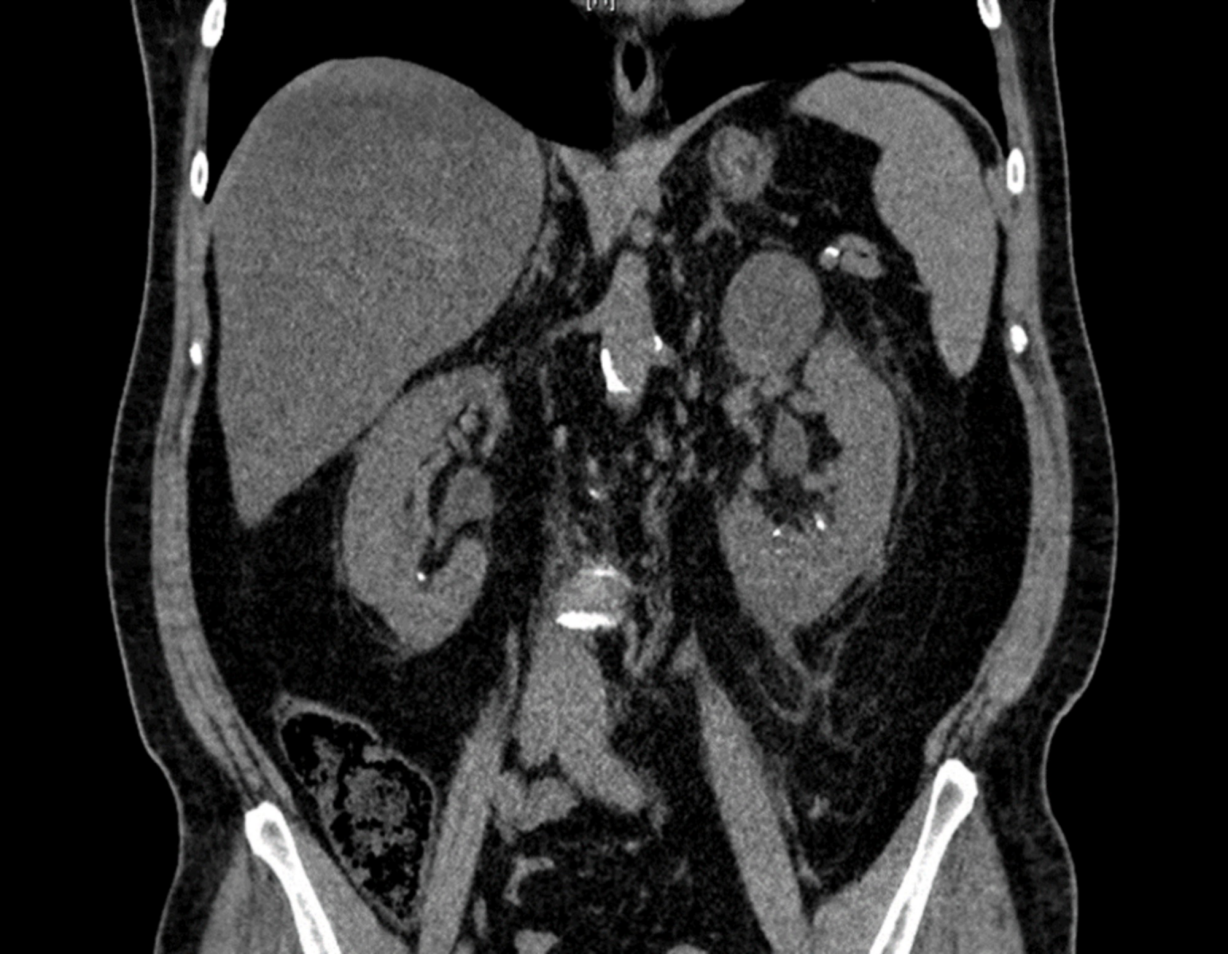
Computed tomography (CT) abdomen/pelvis showing normal kidneys at six months follow-up
